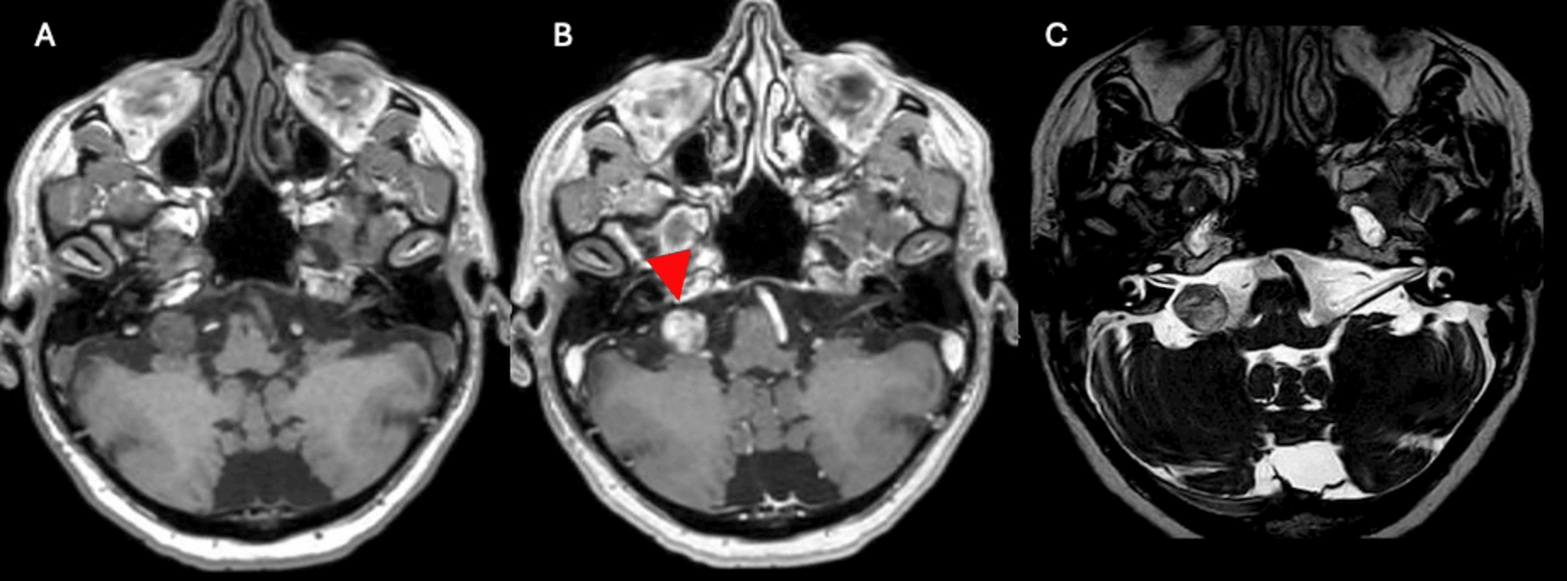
MRI characteristics of the jugular foramen schwannoma after radiation therapy. The lesion is shown on T1-weighted imaging without contrast (A) and with gadolinium administration (B), demonstrating heterogeneous enhancement (B) (red arrowhead). Compared to pre-treatment imaging, gadolinium enhancement appears more pronounced, though this finding should be interpreted with caution. Fast imaging employing steady-state acquisition (FIESTA) sequence (C) reveals a hyperintense signal at the lesion site, which also appears more conspicuous relative to prior imaging.
